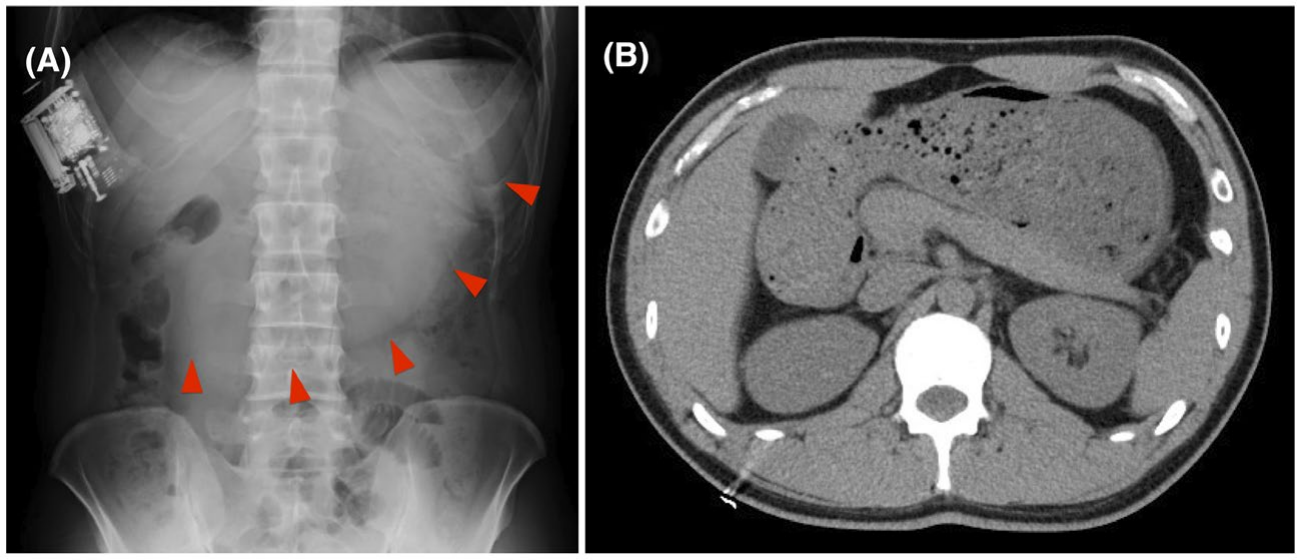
An Abdominal X‐ray radiography was obtained after catheter ablation as the screening test. Red arrows show delayed gastric emptying, therefore this case was diagnosed as acute gastroparesis (AGP) (A). In case that this screening test was inconclusive, the abdominal computed tomography was additionally obtained to diagnose AGP (B)
